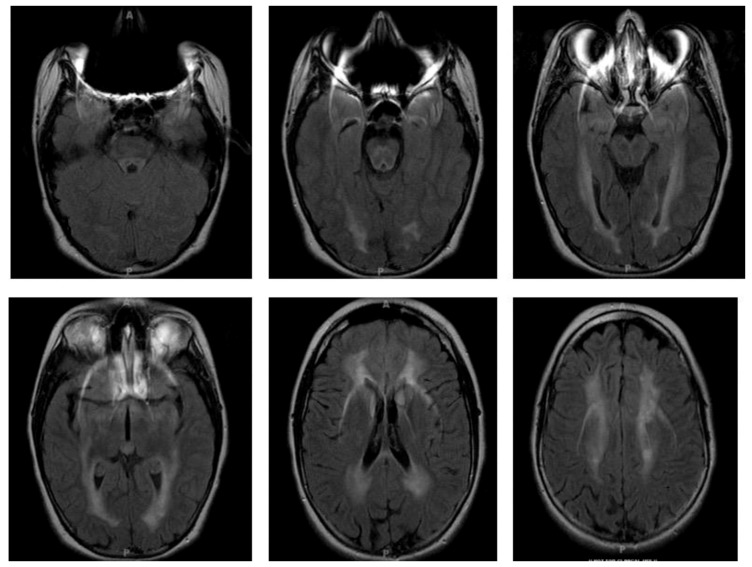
Brain MRI of patient at age 13 (four years after initial presentation), demonstrating global moderate atrophy and leukomalacic changes of the cerebral white matter and deep portion of the cerebellum.
